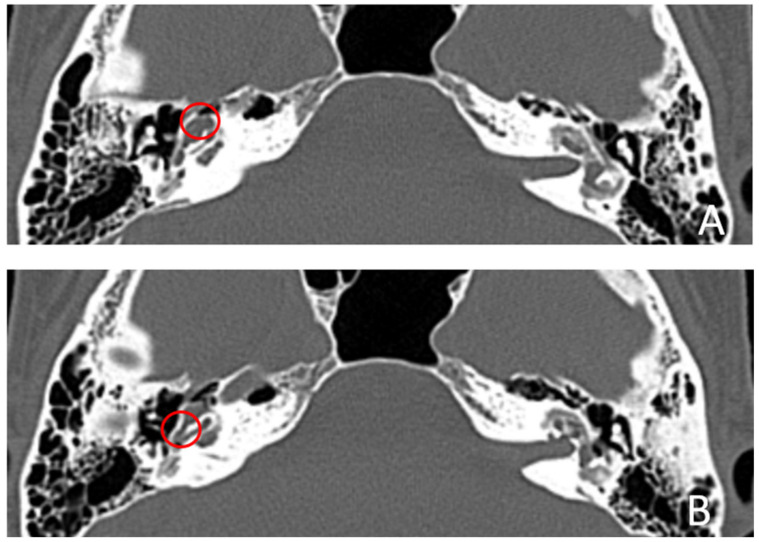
CT images of patient #5 with otosclerosis. (A,B), axial plane. The cavity around the cochlea is only visible on the right side (the circles indicate the contact of the osteorarefation with the cochlea), instead there is only an otosclerotic process without cavity on the left side.
